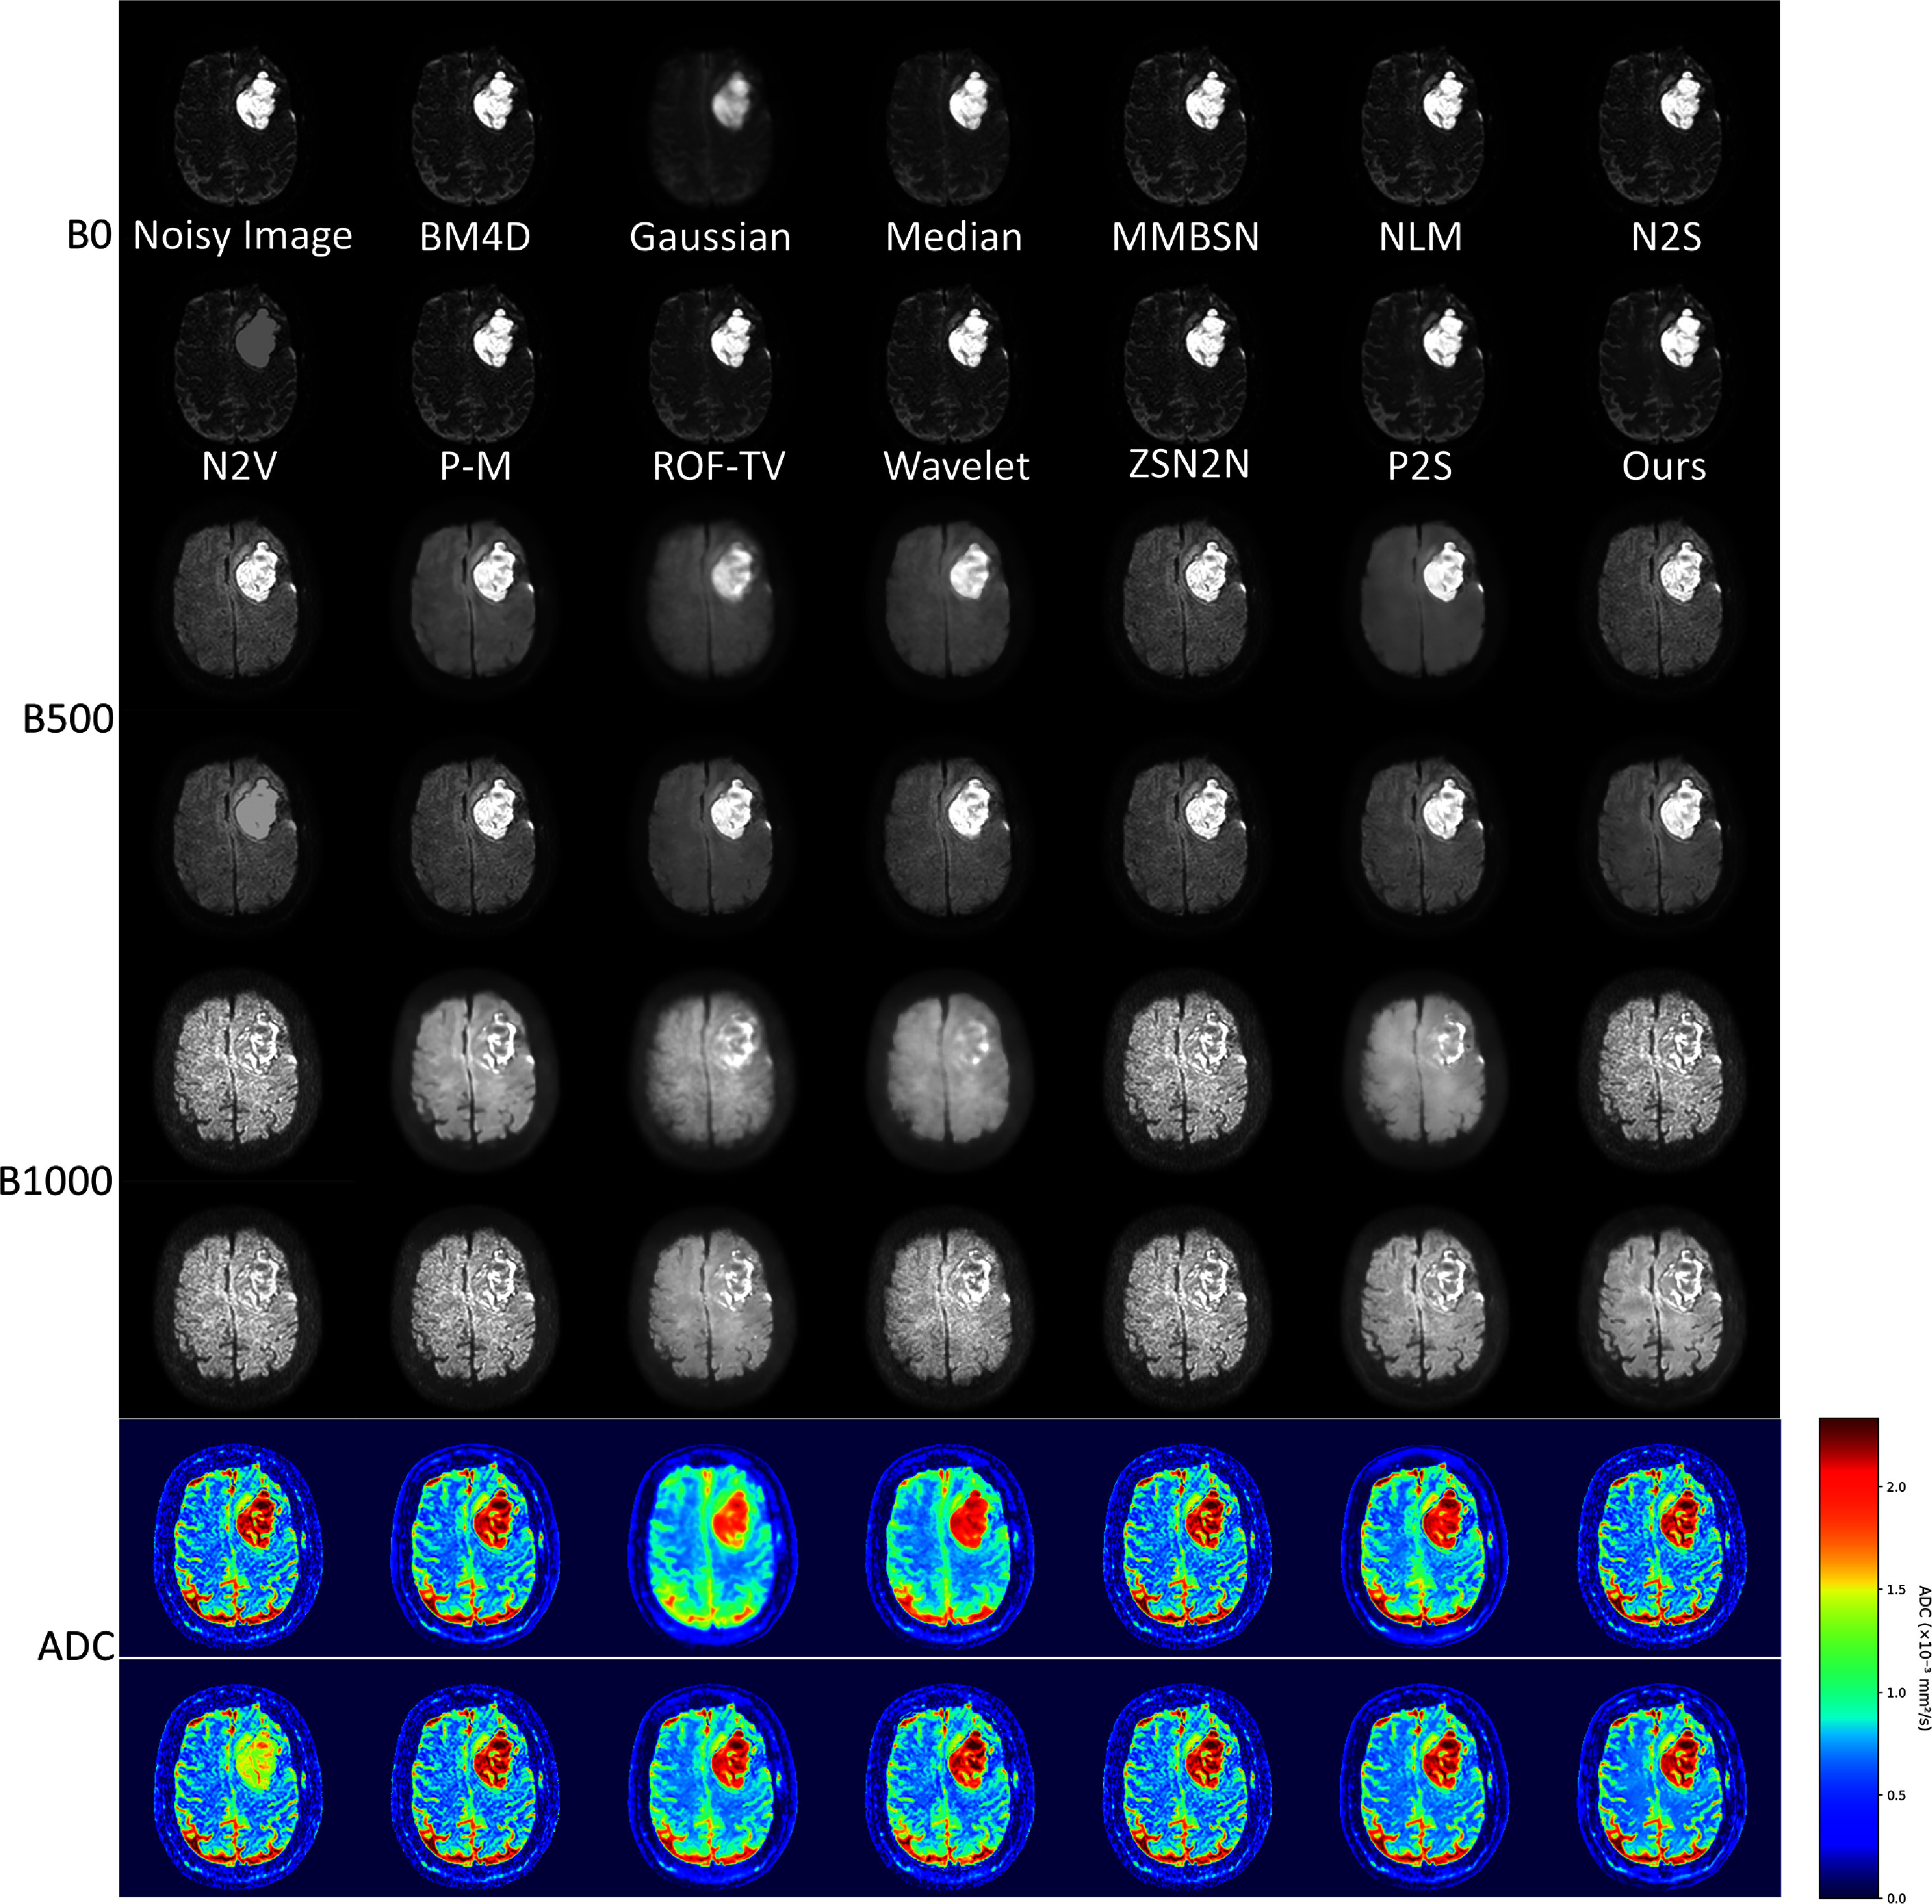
Comparison of brain DWI denoising results. BL-INR demonstrates superior performance in preserving structural details while effectively suppressing noise.
